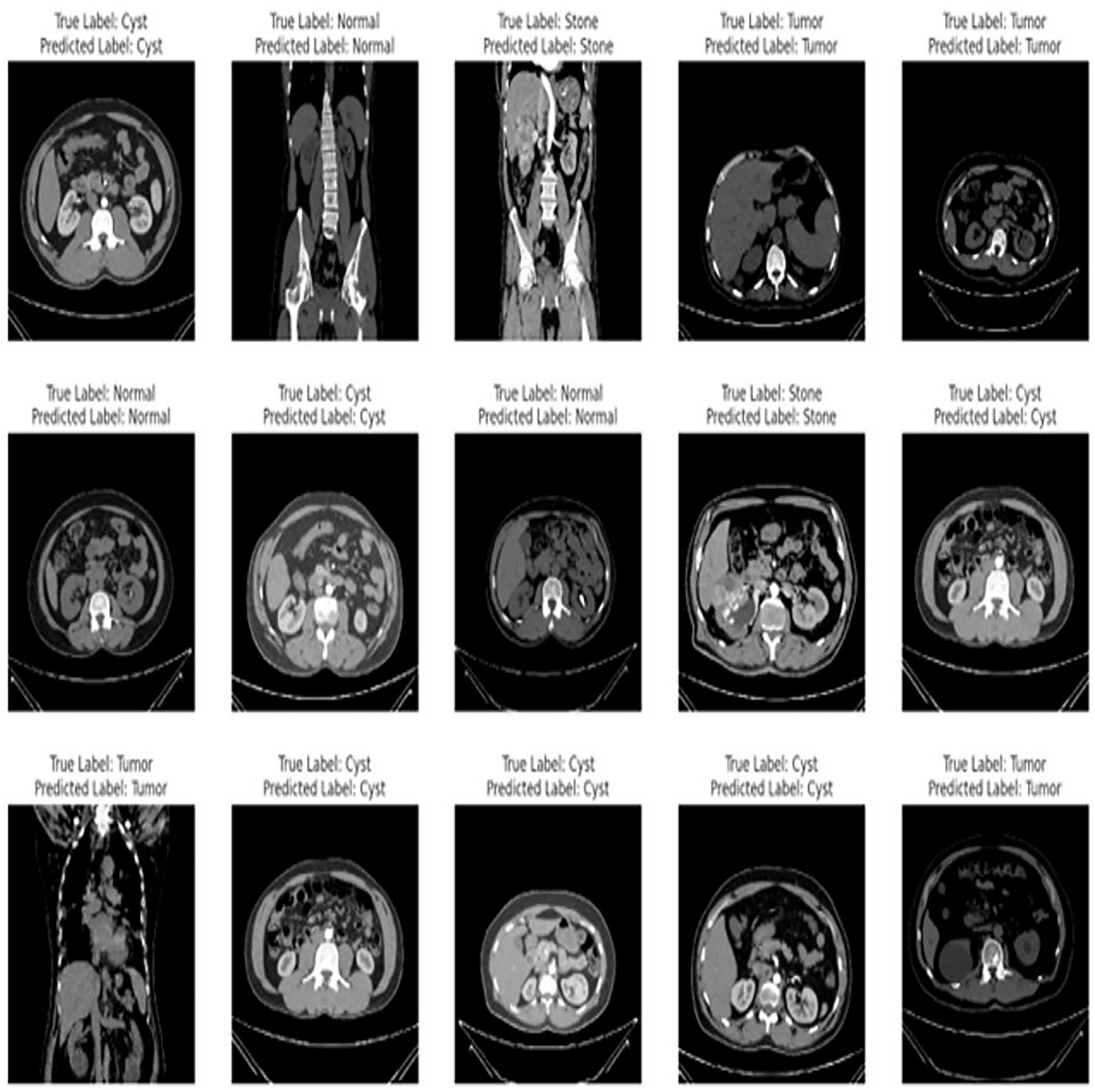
Generalized predictive capabilities of proposed fine-tuned EfficientnetV2B0 on unseen samples of benchmark CT kidney dataset.
